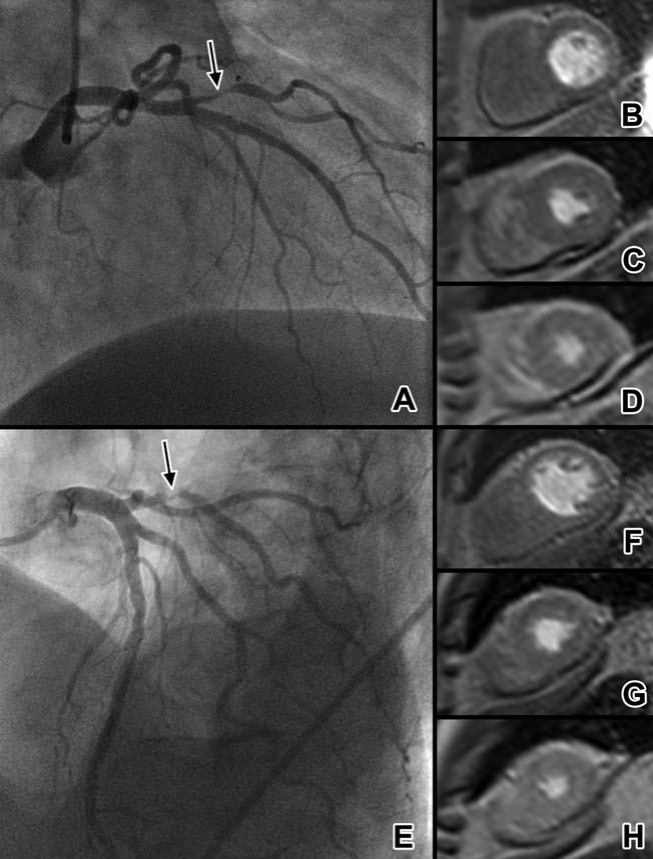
Example of two typical patients with false‐negative MR perfusion. A patient with diagonal branch stenosis (A, arrowed) and absence of a clear perfusion defect on basal (B), mid‐ventricular (C), and apical (D) first‐pass perfusion MR images. SPECT imaging showed a subtle anterolateral perfusion defect. Duke jeopardy score was 2. Another patient with obtuse marginal branch stenosis (E, arrowed) again has no visible perfusion defect on MR (F–H). SPECT was negative in this case, with Duke jeopardy score of 2. MR appears to more commonly miss stenoses which subtend relatively small areas of myocardium.
